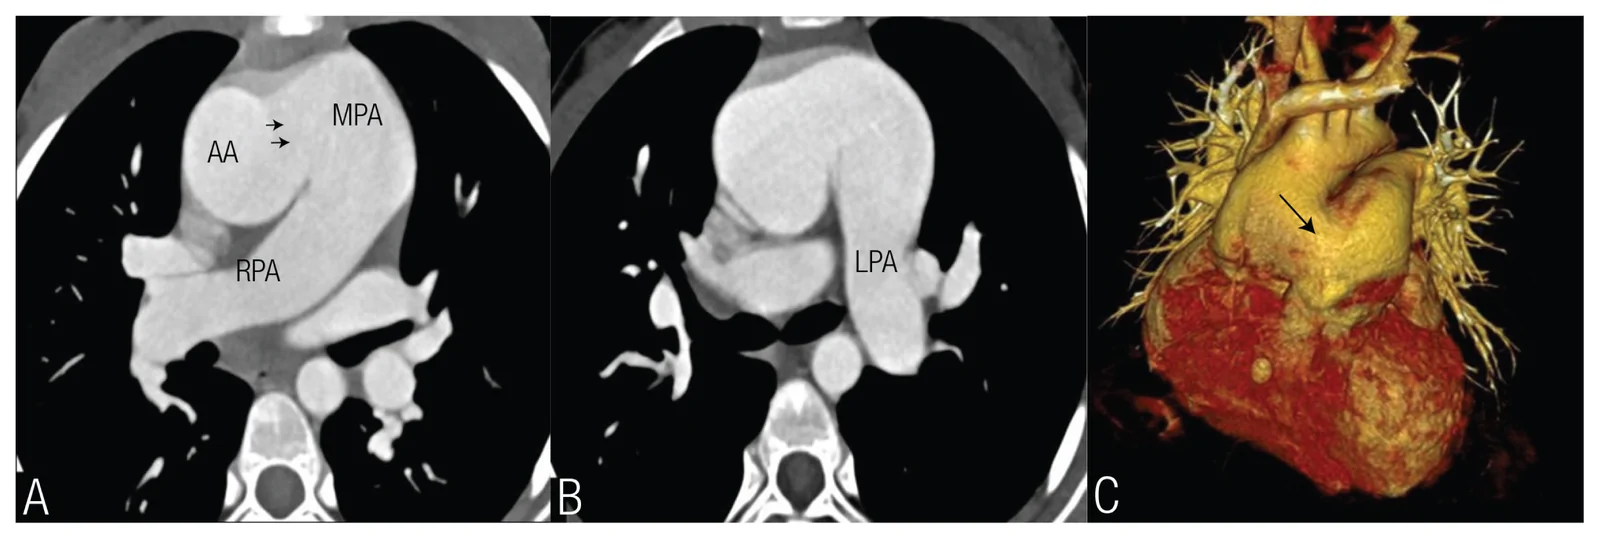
Axial sections of computed tomography angiography at the level of ascending aorta (A and B) showing a large defect (arrowheads) between the ascending aorta and the proximal main pulmonary artery. There is dilated main pulmonary artery, its right and left branch. Volume rendered image (C) showing communication between the ascending aorta and proximal main pulmonary artery (arrow) about 16 mm from aortic root. Left subclavian, left common carotid and innominate artery are arising from the arch of aorta. *MPA = main pulmonary artery; AA = ascending aorta; RPA = right pulmonary artery; LPA = left pulmonary artery*.
